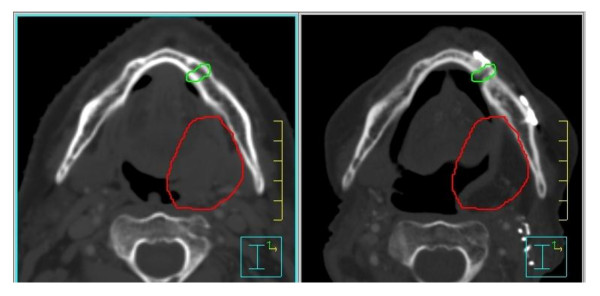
Pre operative treatment distribution planning (left) and fused postoperative CT to assess radiotherapy dose (right).
